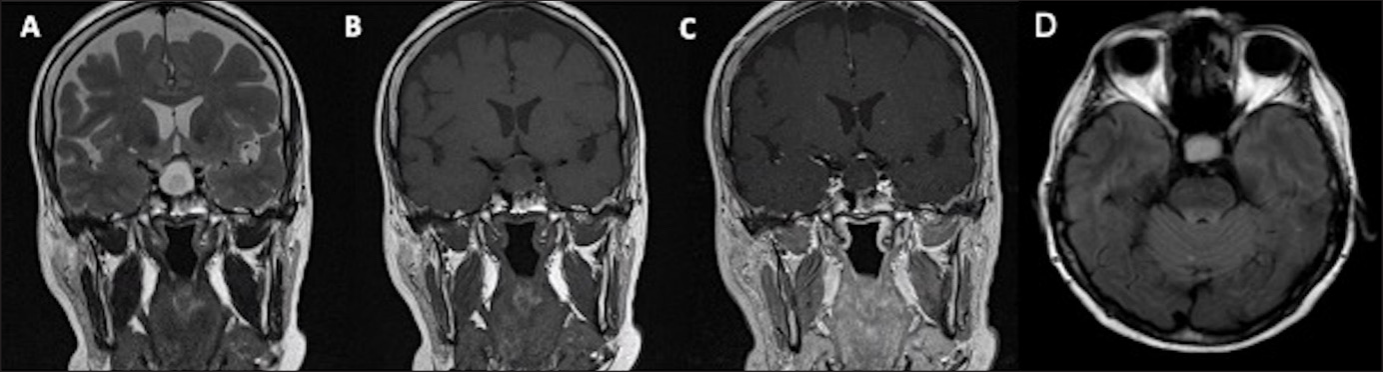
Coronal T2WI (A), coronal T1WI (B), coronal contrast-enhanced T1WI (C) and axial FLAIR (D) images in a 73-year-old female patient show cystic intrasellar and suprasellar lesion with a non-enhancing intracystic nodule hyperintense to surrounding fluid on T1 and hypointense on T2.
